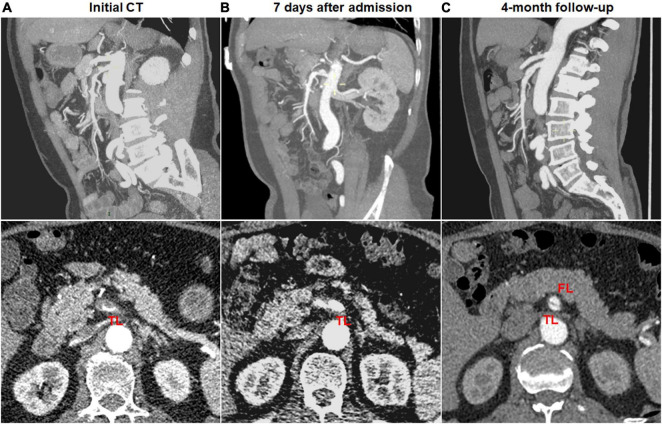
Type change of type III spontaneous isolated superior mesenteric artery dissection on follow-up computed tomography (CT) angiography. (A) Initial CT angiography showed the thrombosed false lumen with ulcer-like projection (ULP) in a 49-year-old man. (B) One week after conservative management, the thrombosis of false lumen was partially resolved and true lumen was slightly dilated. (C) Follow-up angiogram at 4 months after conservative treatment showed double-lumen sign on an axial view and a patent “cul-de-sac” shaped false lumen without re-entry on a sagittal view (type II dissection).
